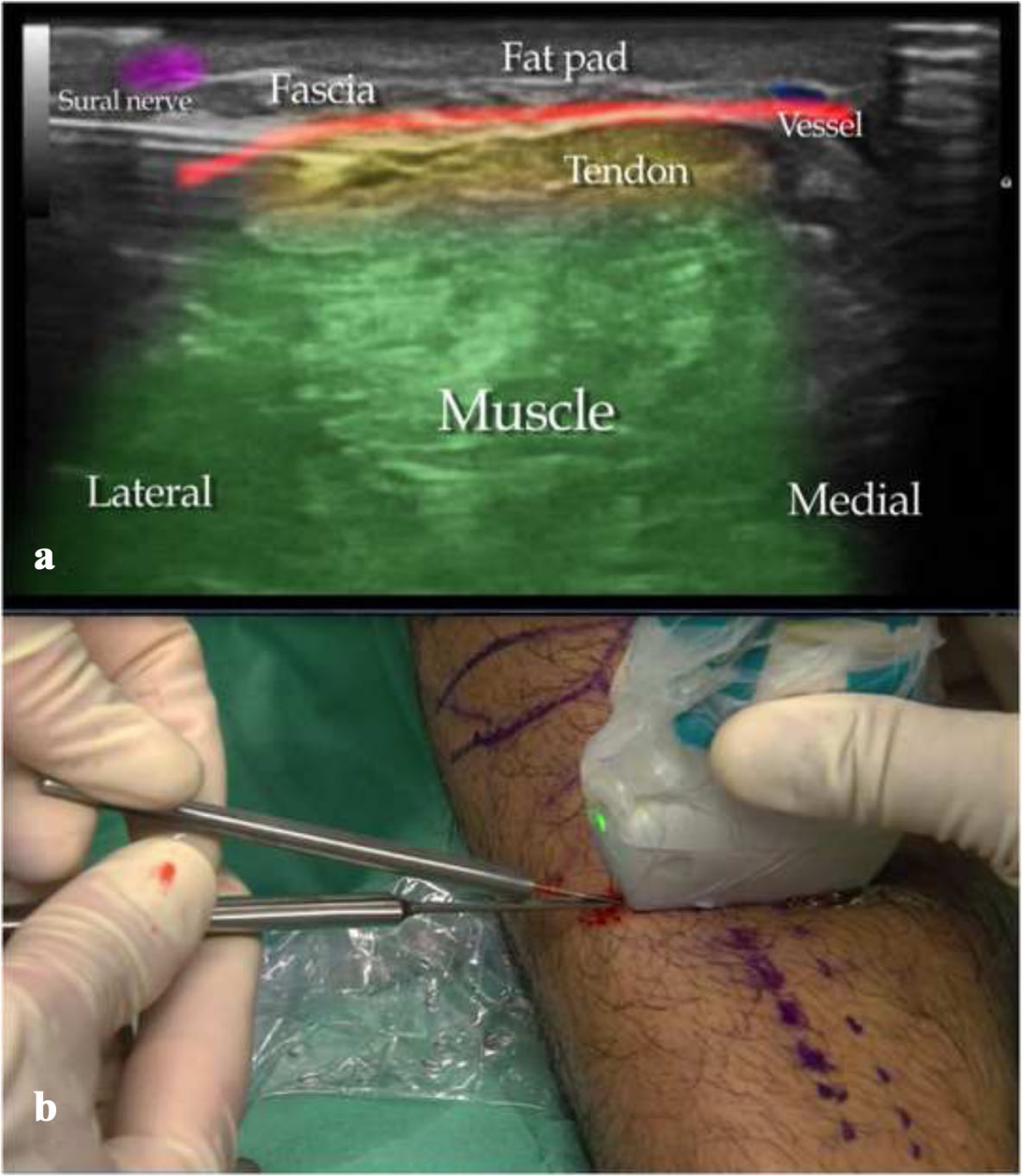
a Surgical technique and b coloured ultrasound-image
